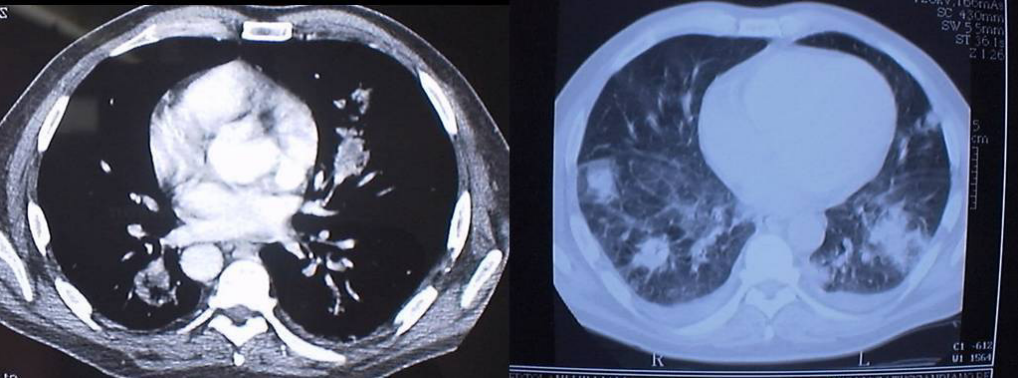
CT scan showing multiple areas of pulmonary infarction.
